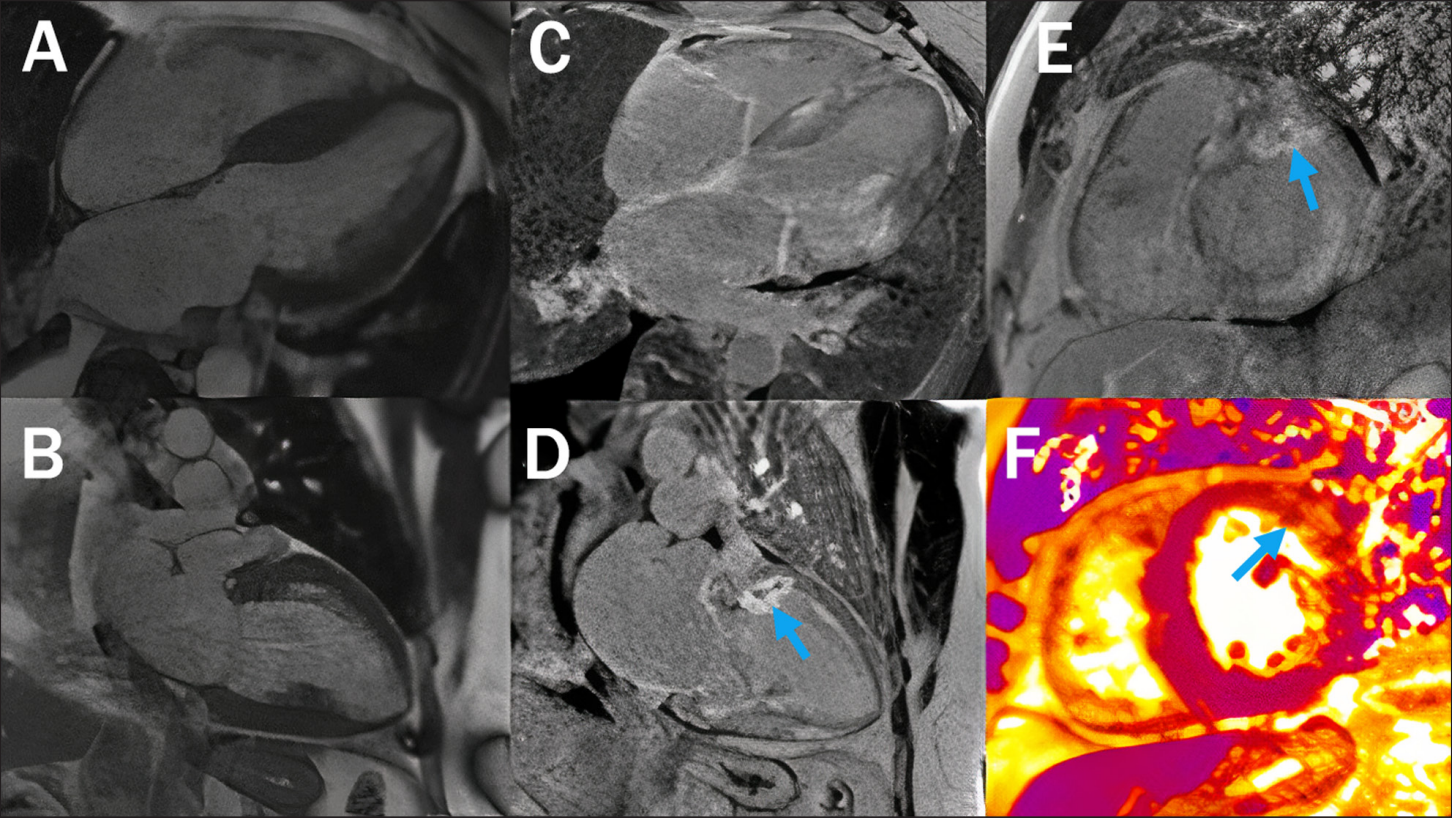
Cardiovascular magnetic resonance imaging showed (A, B) diffusely increased left ventricular wall thickness with (C–E) diffuse patchy late gadolinium enhancement (LGE) in the left ventricle and both atria consistent with cardiac amyloidosis. (D, E, arrow) There also is dense focal transmural LGE in the basal anterior and anterolateral wall with microvascular obstruction at its center and myocardial edema noted on T2 mapping images (F, arrow) that suggest acute myocardial infarction in a ramus or diagonal artery distribution. Image enlarged using letsenhance.io.
